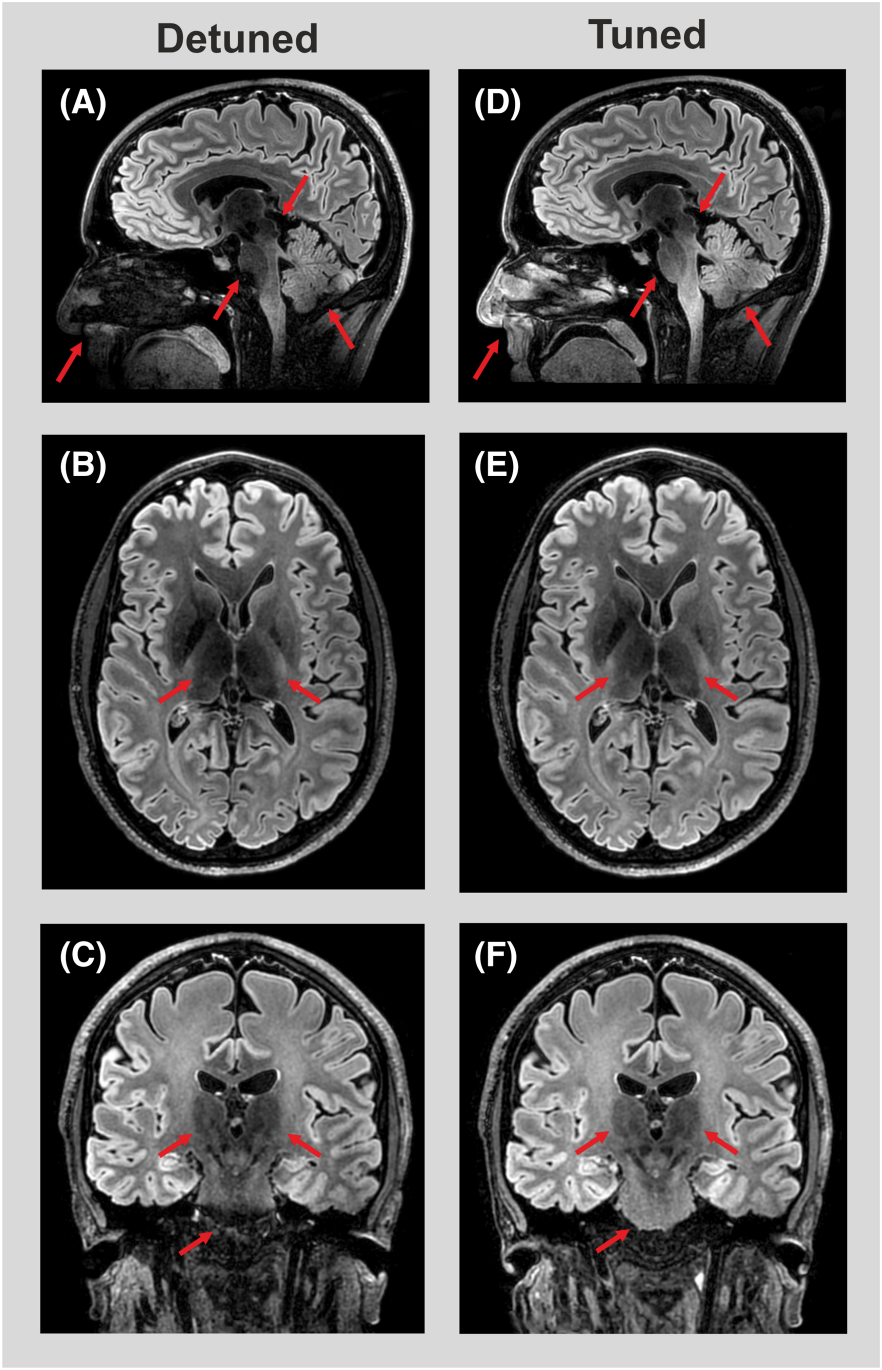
High‐resolution FLuid‐Attenuated Inversion Recovery (FLAIR) images collected with the eight‐channel parallel radio frequency (RF) transmission (pTx) head‐coil using the 32‐channel receiver array (A‐C) in combination with a detuned transmitter compared with all 40 channels, which include the eight tuned transmit channels (D‐F). More homogeneous receive performance is observed when the signal of the eight transmit channels is incorporated, and higher signal‐to‐noise ratio (SNR) is observed outside the brain
